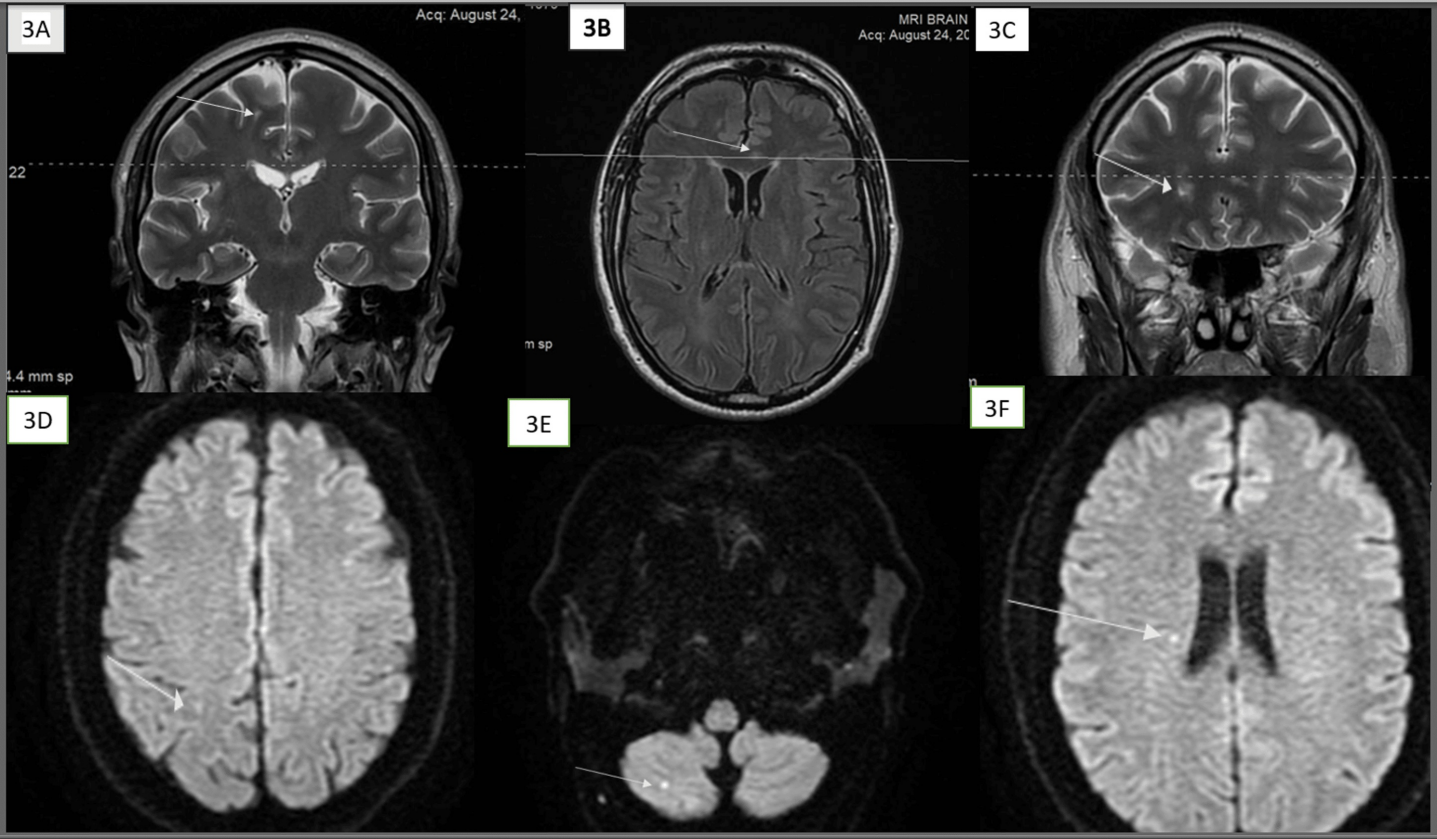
Brain MRI in August 2022 showing the development of a new set of lesions (3A) A new T2 hyperintense white matter lesion in the right superior frontal gyrus (arrow). (3B) Another new hyperintense lesion in the genu of the corpus callosum seen on the axial FLAIR image. (3C) The same image in 3B shown on T2-weighted coronal images. (3D) Another white matter lesion in the right parietal subcortical white matter, showing diffusion restriction. (3E) Yet another white matter lesion in the right cerebellar hemisphere showing diffusion restriction. (3F) One more new lesion in the right periventricular white matter showing diffusion restriction FLAIR: fluid-attenuated inversion recovery
